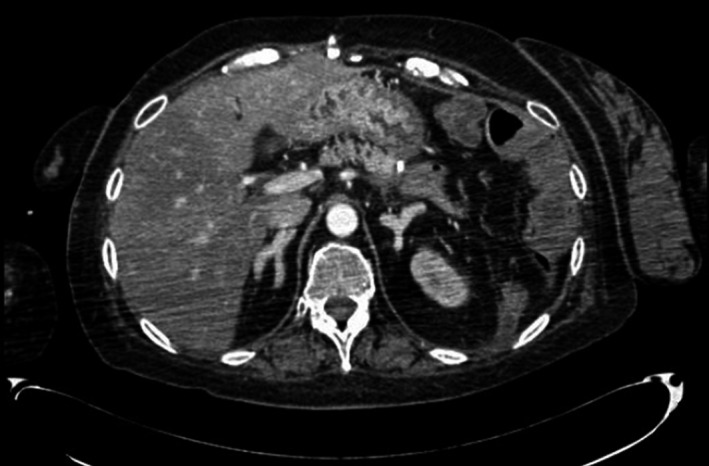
Abdominal CT scan showing bilateral adrenal hyperplasia secondary to the chronic stimulatory effect of ectopic ACTH secretion.
